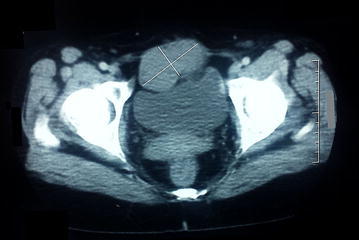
Computed tomography showing the abdominal wall recurrence
